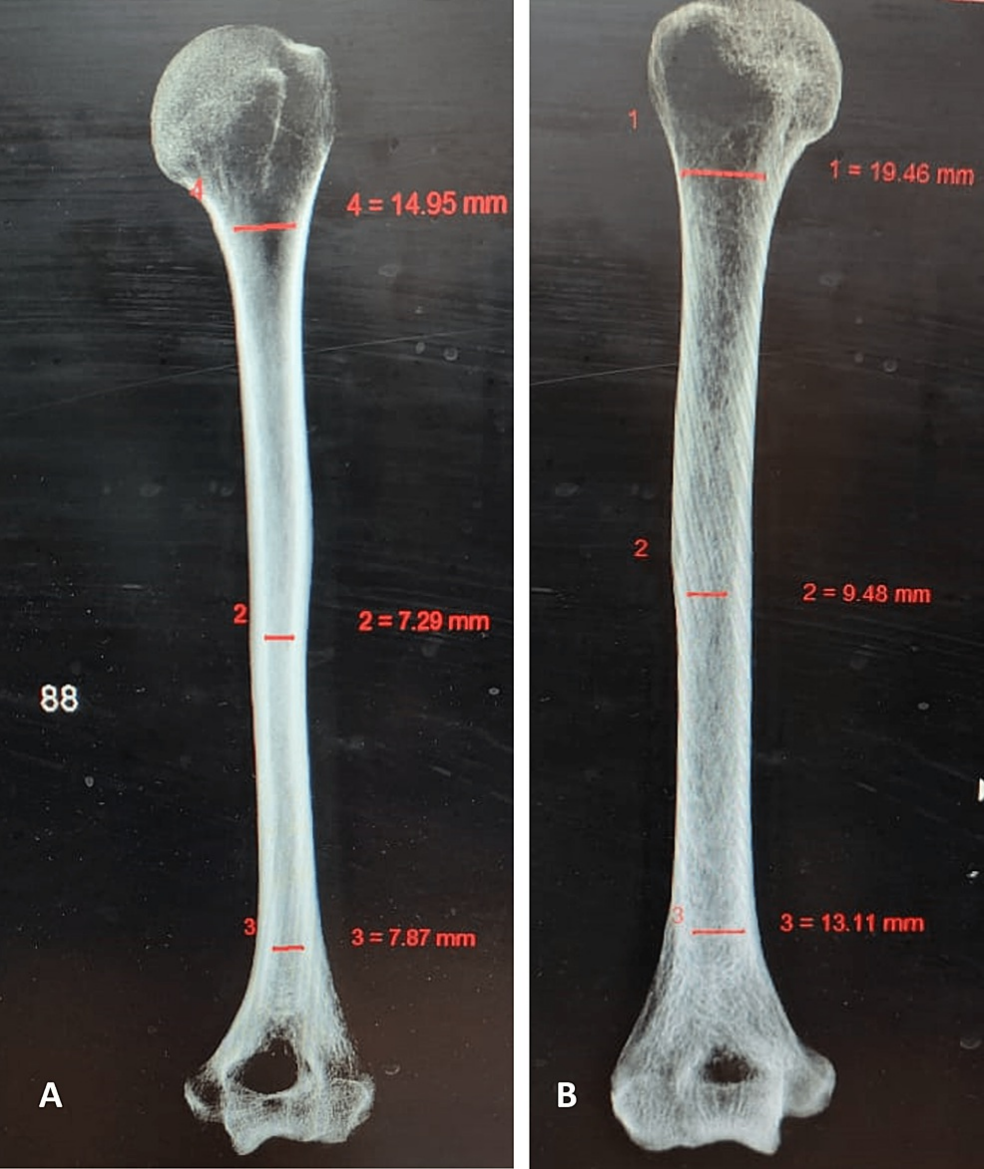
Radiographs of humeri bones show the width of medullary canal in bone with (A) and without (B) supratrochlear foramen.
